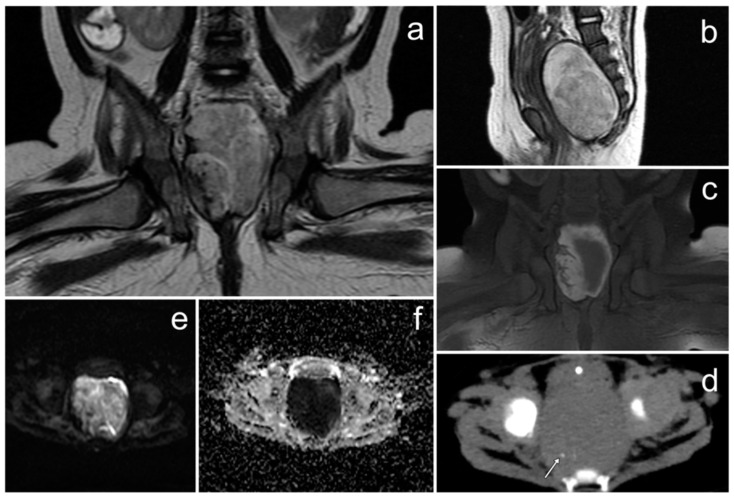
MRI of pelvic neuroblastoma (a–c,e,f). Small punctate calcification in tumor on CT scan (d, arrow). (a,b)—FSE/T2; (c)—post-contrast FSE/T1 FS; (e)—DWI; (f)—ADC map.
